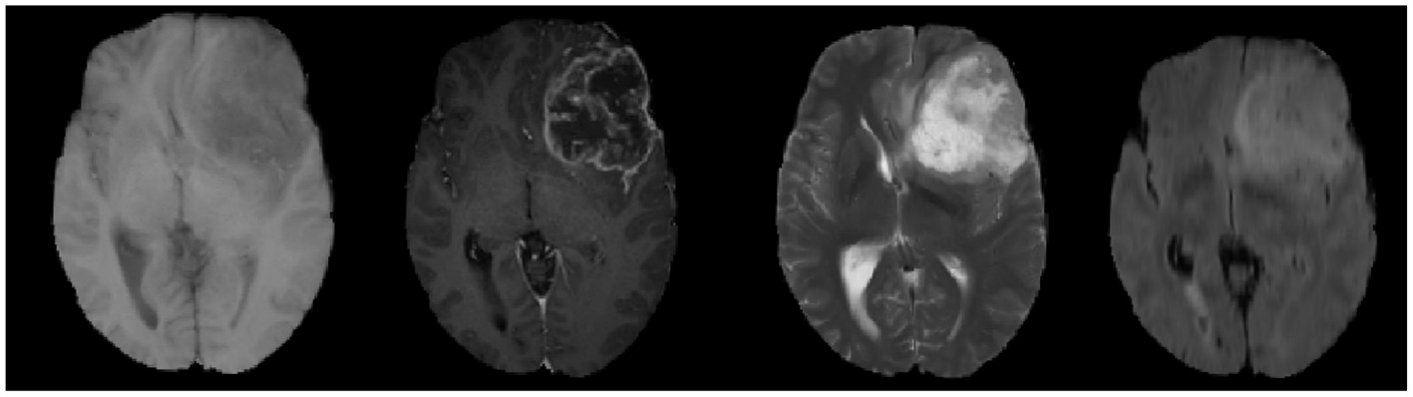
MRI images of four different modalities.
